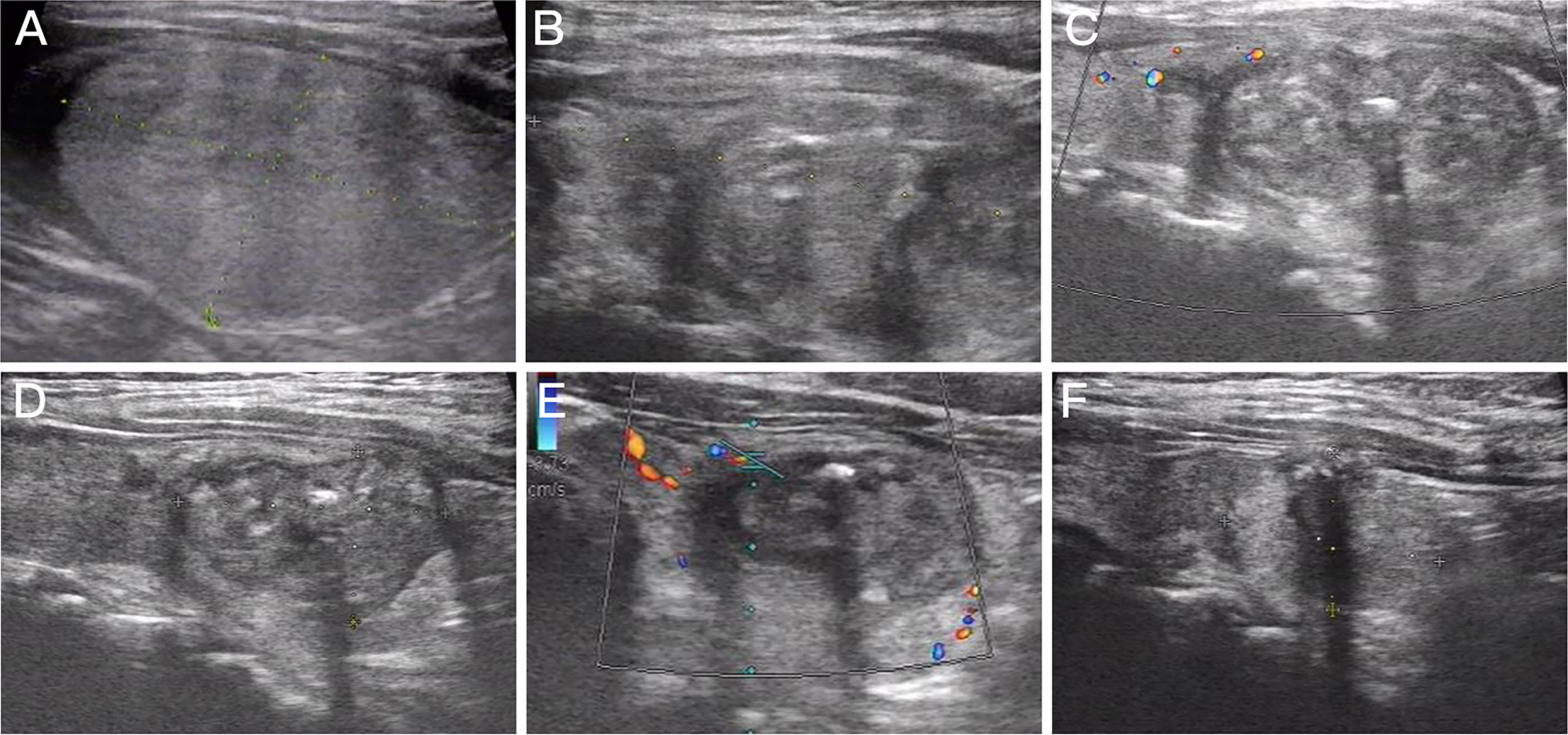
Imaging of a 63-year-old woman with Bethesda IV nodules who underwent microwave ablation (MWA). (A) An isoechoic solid thyroid nodule was detected in the left lobe with an initial volume of 14.29 ml. (B) Ultrasound examination showed enlarged ablation area (20.16 ml) immediately after MWA. (C–F) The volume of the ablation area was 12.39, 6.33, 3.13, and 2.04 ml at 1, 3, 6, and 12 months after MWA, respectively.
